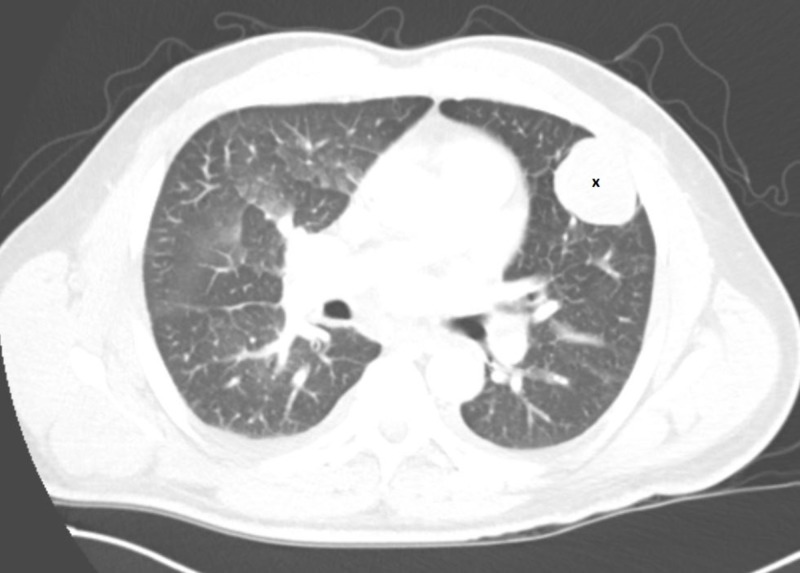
Computed tomography imaging of the lungs revealing large 4.5 cm x 5 cm circumferential pleural-based mass (x) consistent with metastatic sarcoma.
